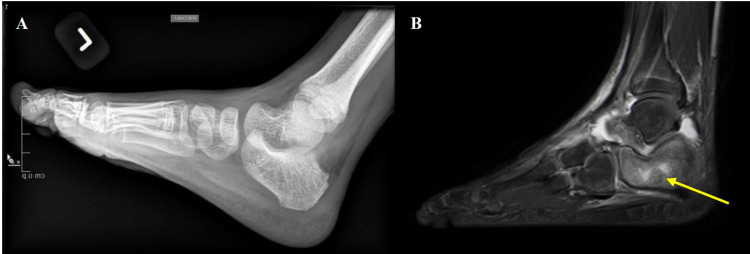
(A) Lateral radiograph of the left foot and ankle. (B) T2 weighted MRI of the foot and ankle. Patient was admitted for limping and heel pain. Plain radiograph is normal. MRI demonstrates extensive edema of the calcaneus, soft tissue inflammation, and fluid in the subtalar joint consistent with osteomyelitis/septic arthritis.
